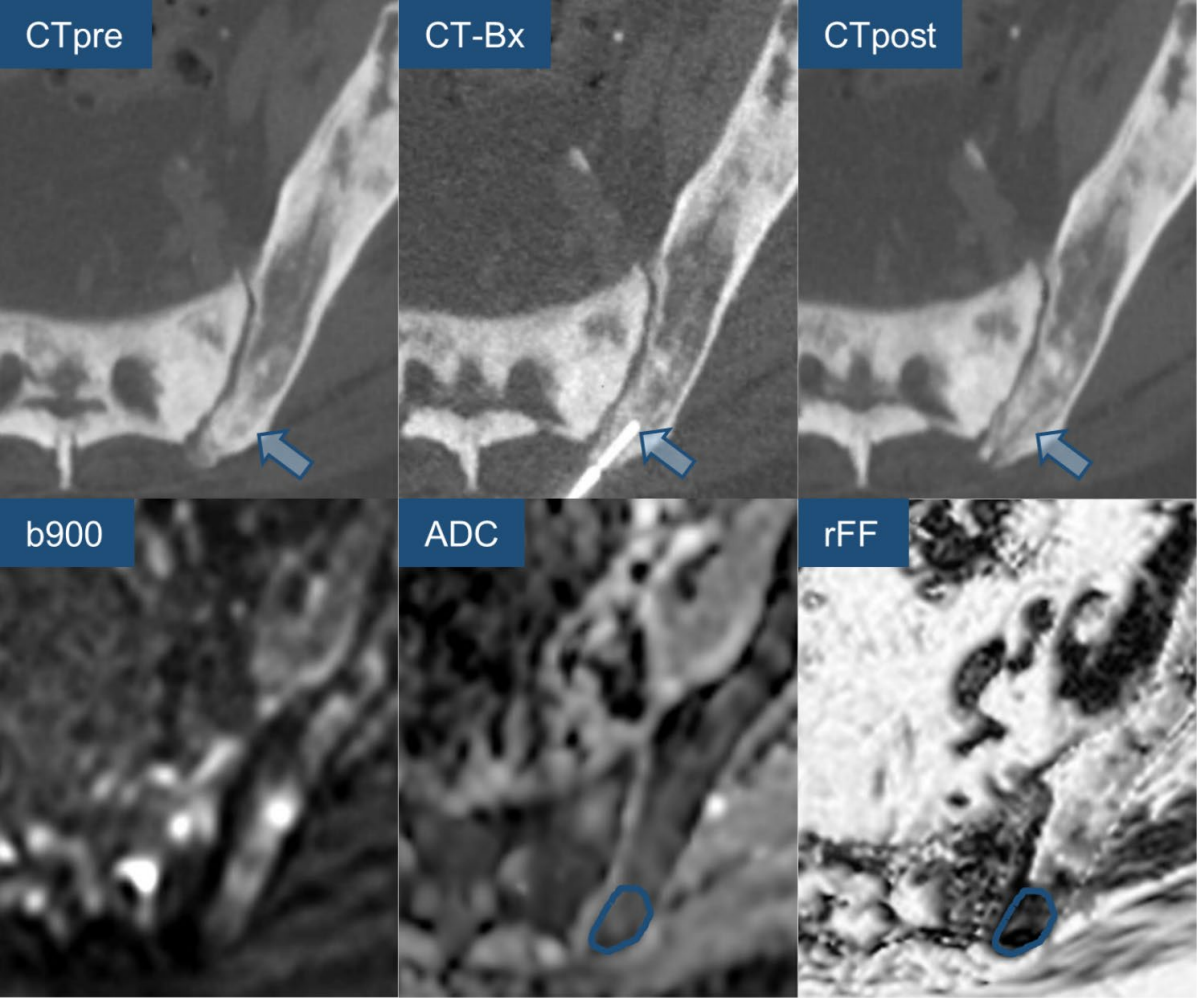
CT-guided bone biopsy in a 86-year old prostate cancer patient, CT before biopsy (CTpre) displays diffuse sclerosis, the chosen target area according to the b900 DWI, ADC and rFF is indicated by the arrow, the biopsy-CT (CT-Bx) and post-interventional control spiral (CTpost) show biopsy needle and biopsy tract in the target area, note how the majority of the sclerotic pelvic bone displays high signal on the rFF image
